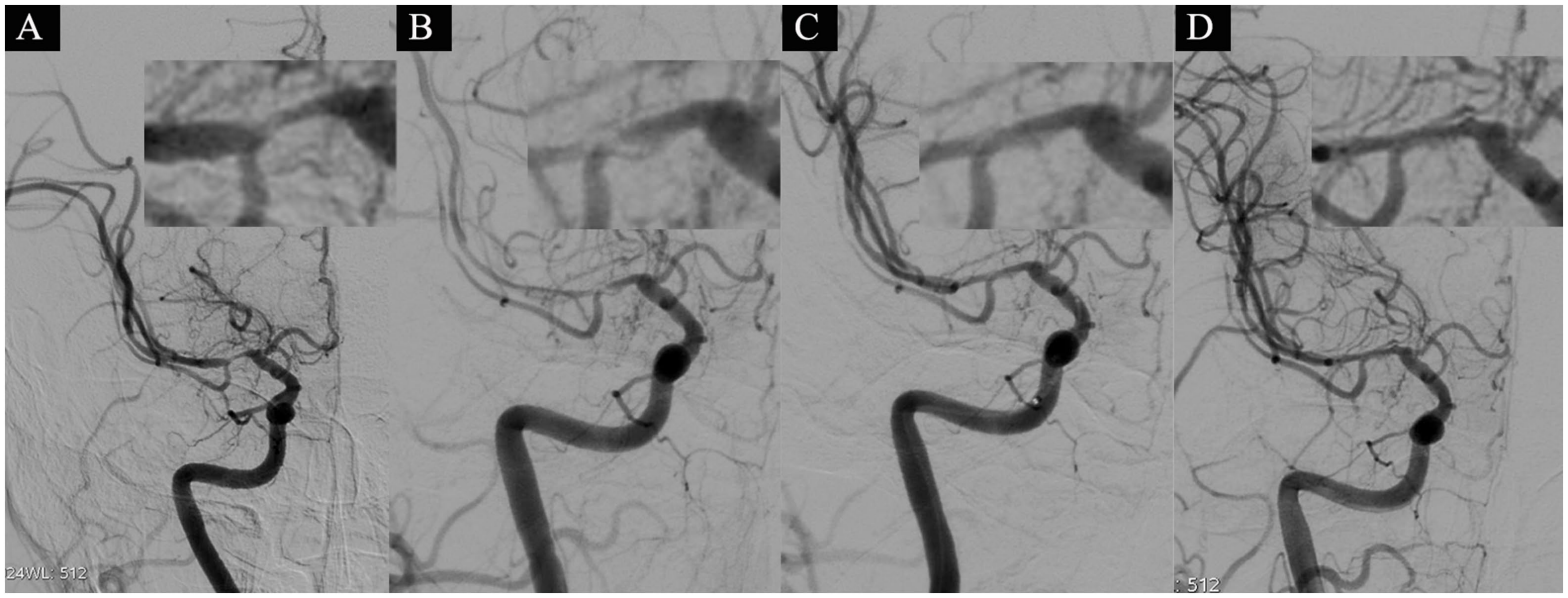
A 64-year-old female presented with acute-onset left-sided limb numbness, weakness, and slurred speech, prompting admission on March 7, 2020. The NIHSS score at admission was 5 (facial palsy 2, sensory deficits1, language impairment 1, dysarthria 1). Head MRI revealed an acute ischemic stroke adjacent to the right lateral ventricle. On March 25(after 19 days of stroke onset), cerebral angiography revealed severe stenosis with a distal diameter of 2.0 mm, a narrowest segment of 0.5 mm, and a stenosis degree of 75% (A). Subsequent dilation with a 1.5 × 15 mm balloon led to an arterial dissection (B). A slow infusion of 5 mL of tirofiban was administered through the artery, and after a 15-min observation, the dissection showed improvement, achieving eTICI grade 3 blood flow (C,D).
